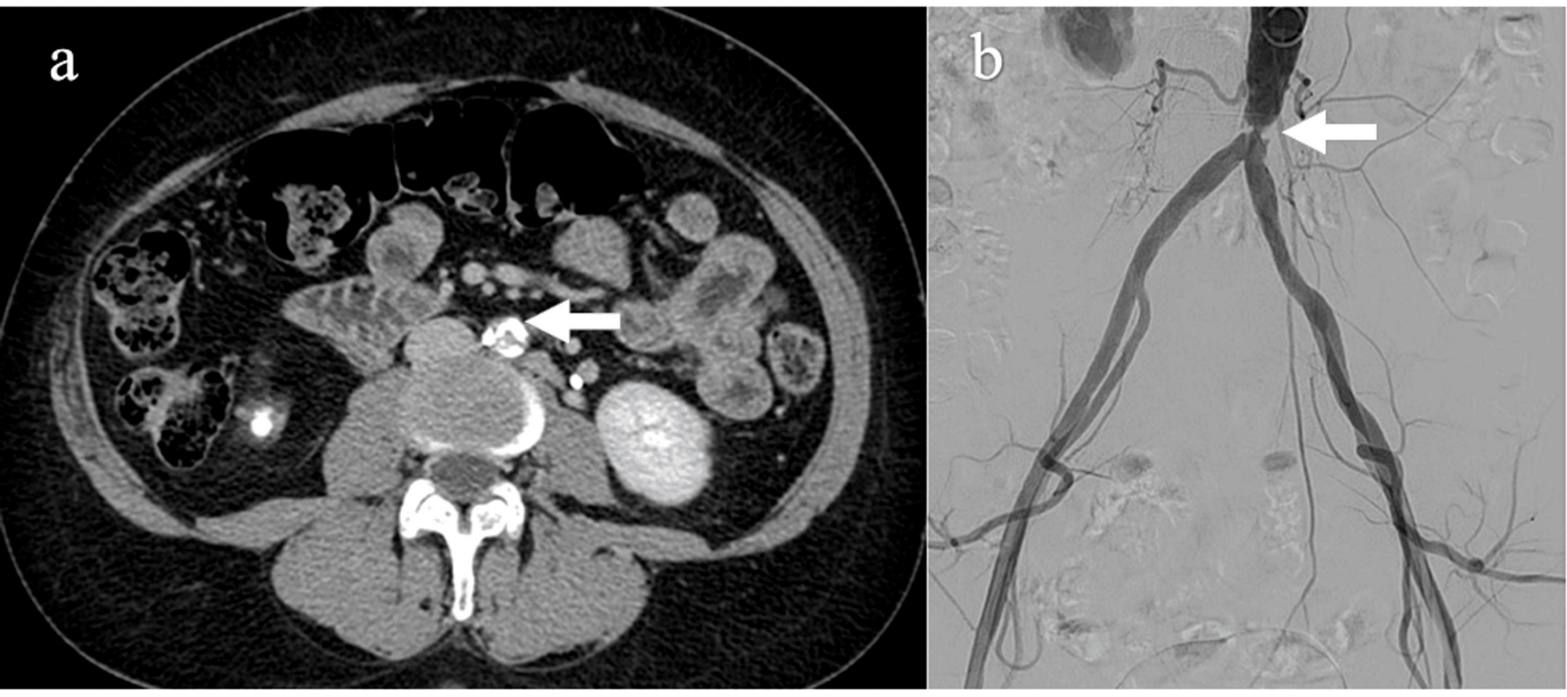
(a) Angio-CT scan, stenosis of the infrarenal abdominal aorta with severe calcifications (arrow); (b) angiography, stenosis of the distal abdominal aorta (arrow).
